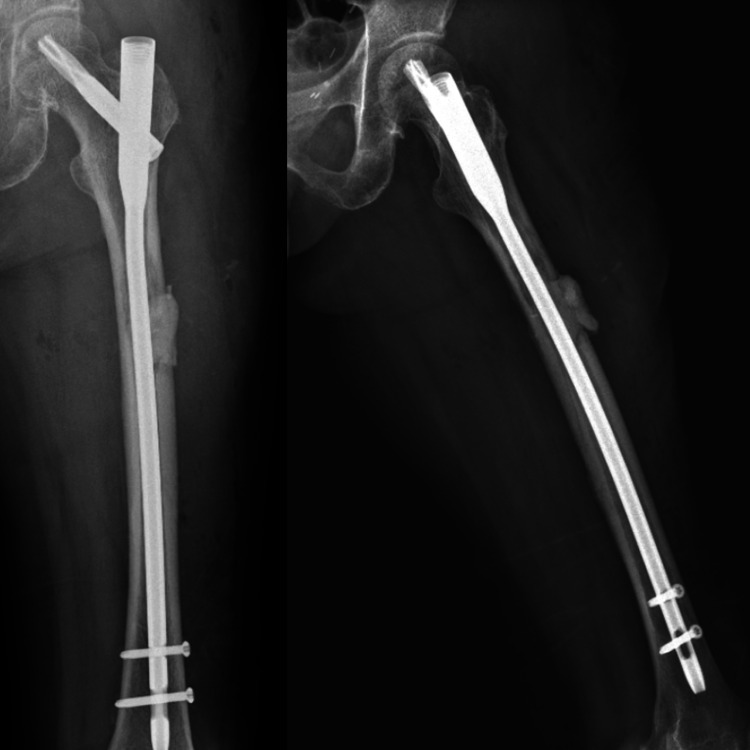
Post-operative plain radiography showing left proximal femur nail implant
